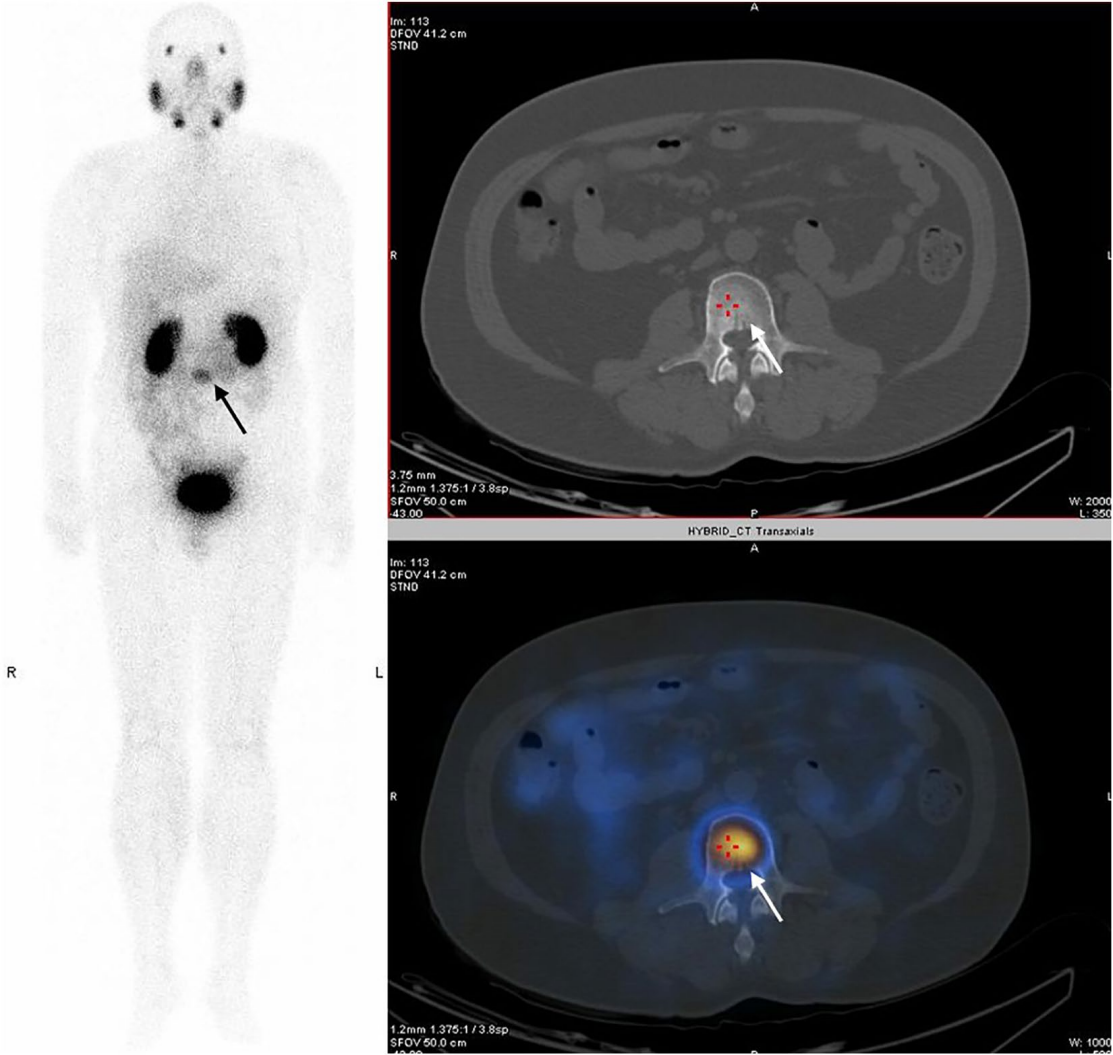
Maximum intensity projection (left), CT (top right) and fused (bottom right) ^99m^Tc-HYNIC-PSMA SPECT/CT images of a 77-year-old patient with a PSA of only 0.56. A L3 vertebral metastasis was detected (arrowed) in fused image, but no perceptible lesion was found on CT anatomical imaging.
